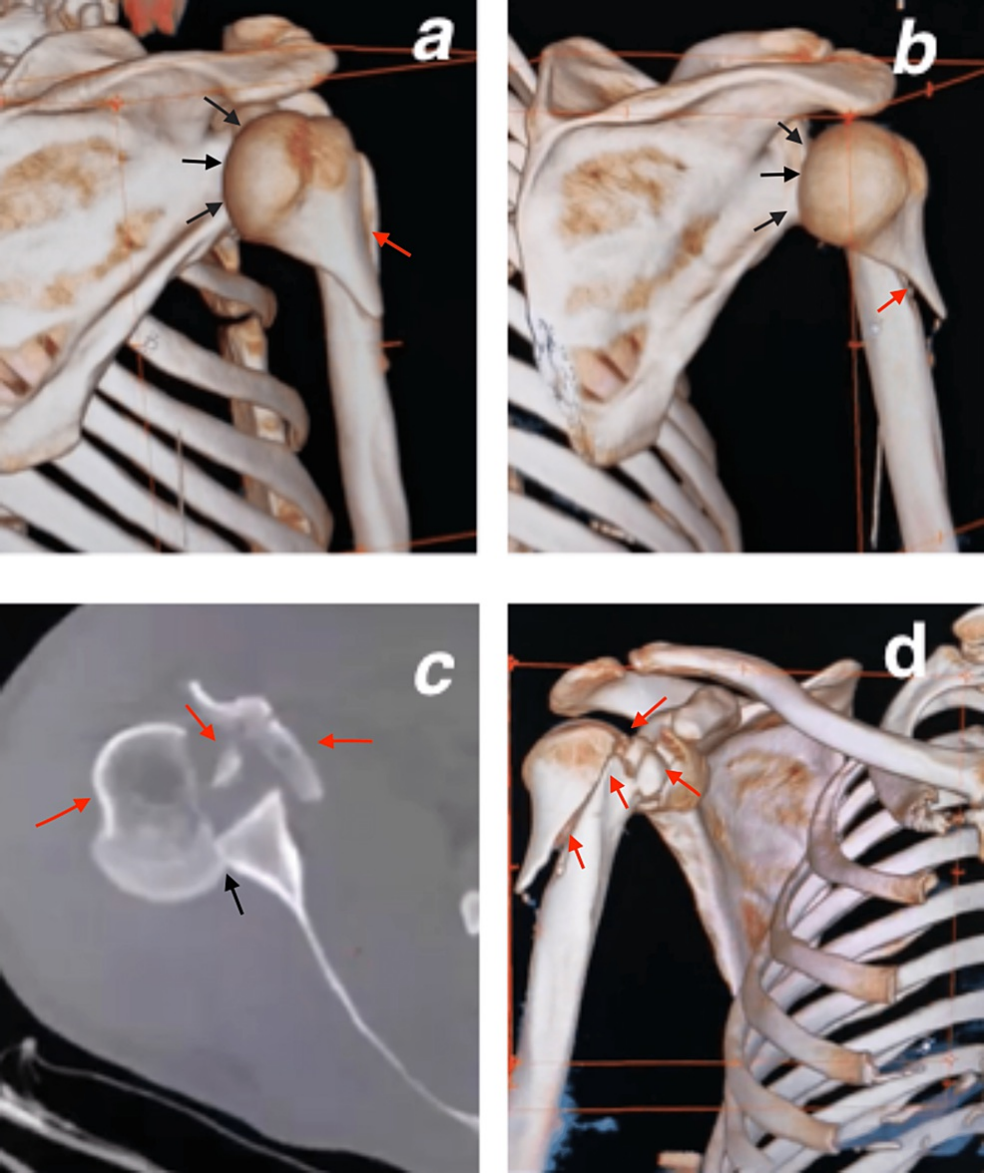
CT scan of the right shoulder after the trauma shows the three-dimensional reconstruction slices (a-d), axial slice (c), and a complex three-part posterior fracture (red arrow) with dislocation (black arrow) of the right shoulder.
